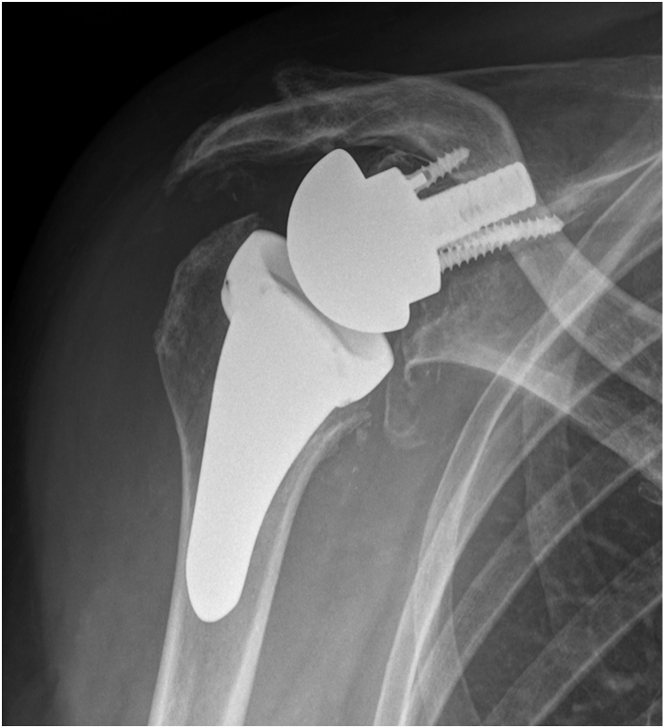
An anteroposterior radiograph of the right shoulder demonstrating loosening of the glenoid baseplate, glenoid dislocation, and possible glenoid fracture.
